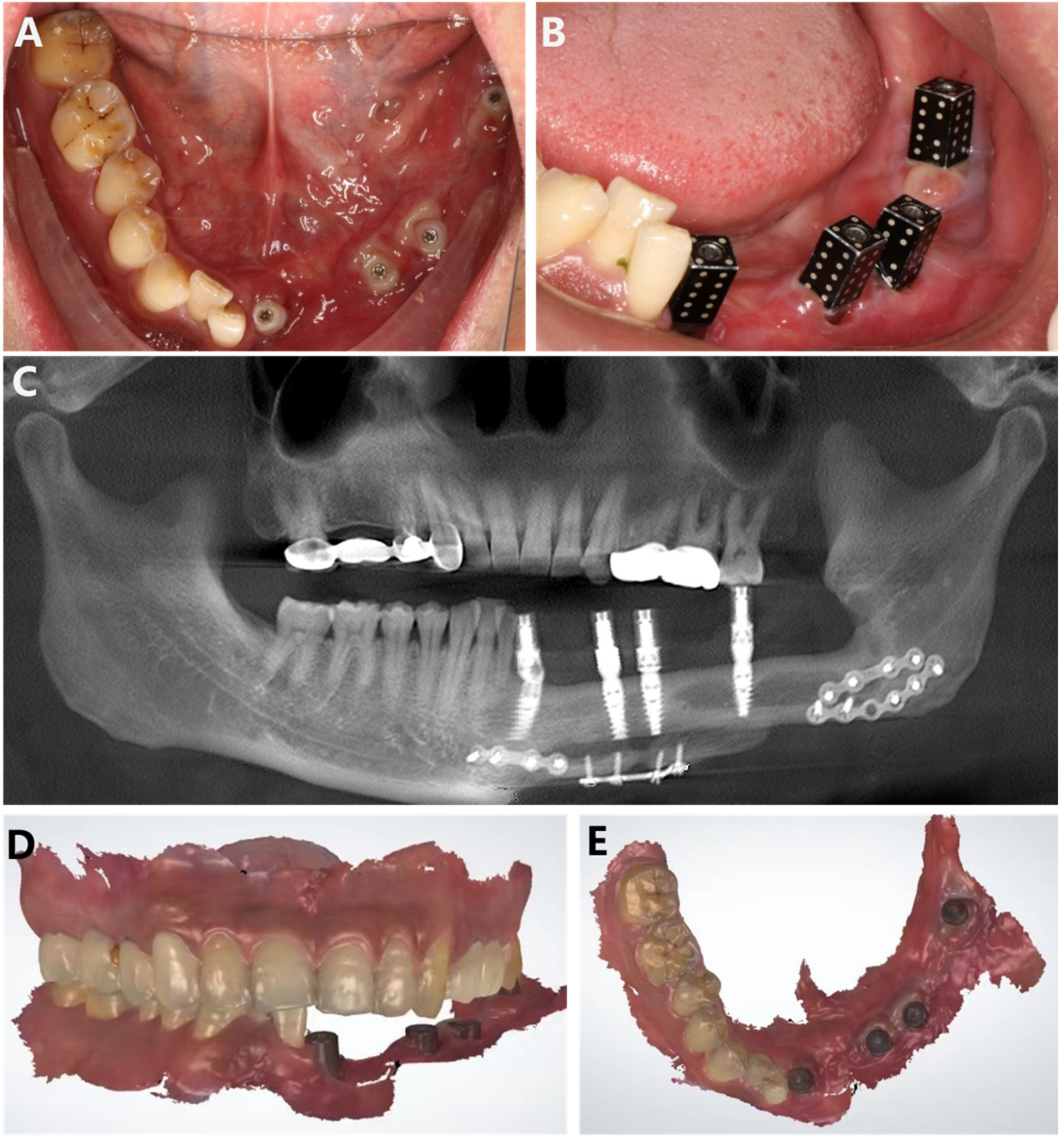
(A) Intraoral photo 2 months post-gum grafting, showing good healing of the ADM. (B) Placement of ICamBodies for ICamPosition. (C) CBCT imaging taken after placing the ICam4D extraoral scanning accessory. (D,E) Intraoral scan data.
